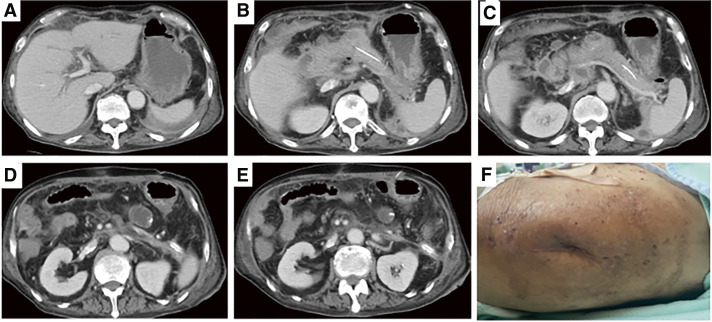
CT images and wound findings on POD day 115. (A–E) Encapsulated necrotic tissue has shrunk and fluid retention has improved. (F) Complete closure of the wound has been achieved. CT, computed tomography; POD, postoperative day
